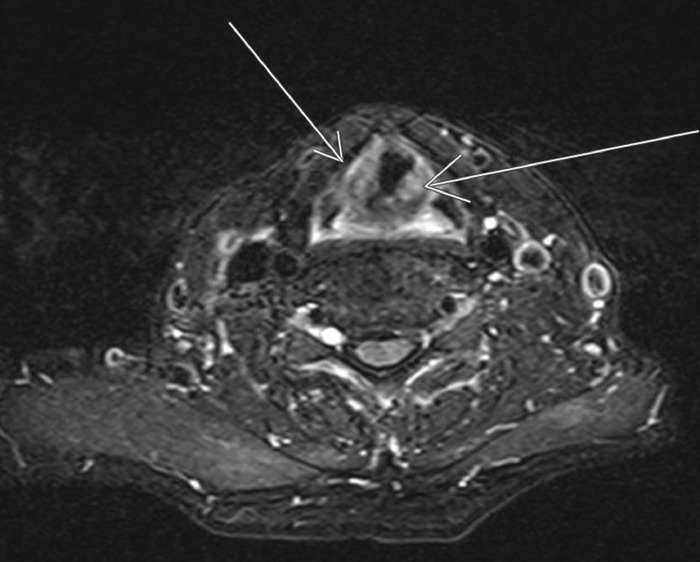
T2 noncontrast magnetic resonance imaging of soft tissue in the neck is positive for heterogeneous edema (arrows) of the vocal cords.
